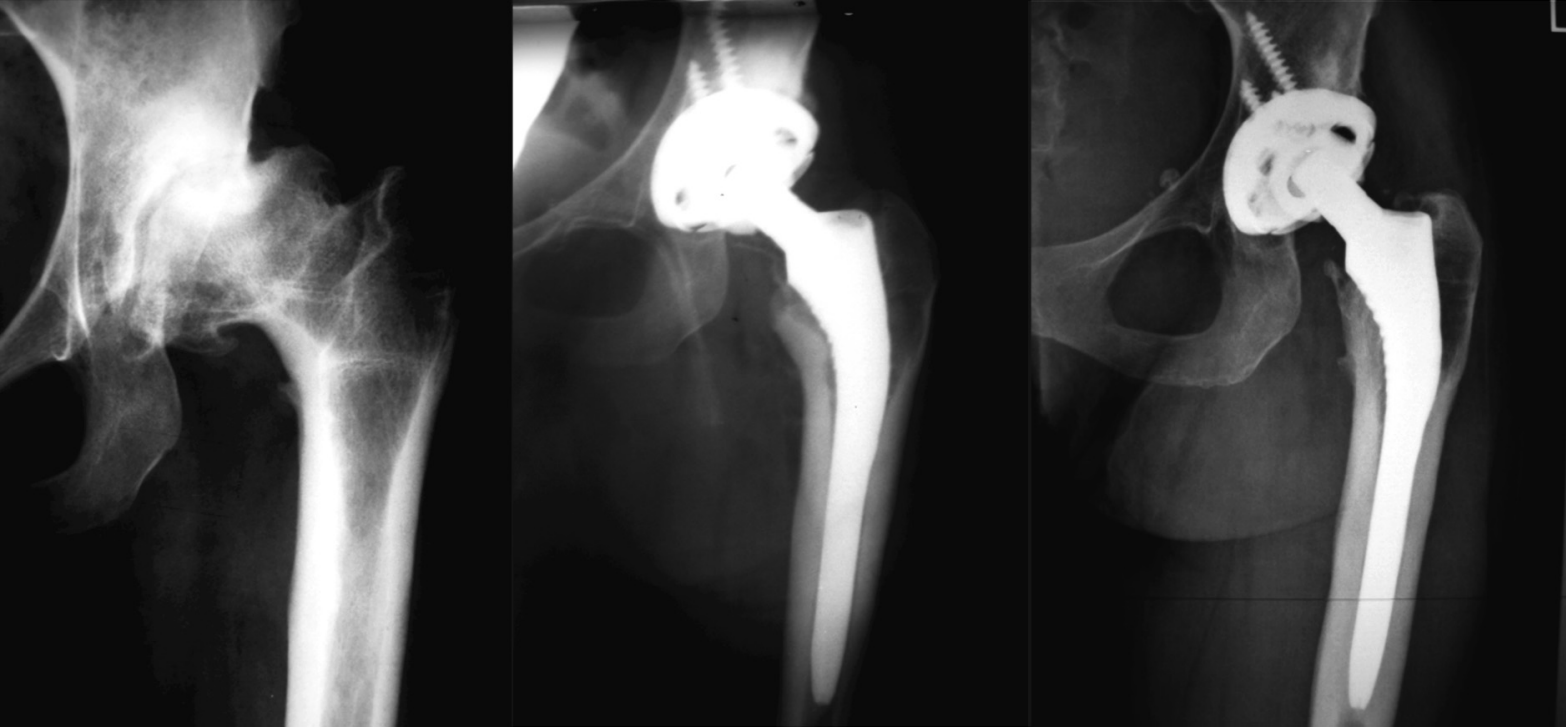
26-year-old woman with severe secondary OA of the left hip, femoral deformity, and varisation osteotomy. Radiographs (left to right) preoperatively, and 1 year and 15 years postoperatively.
